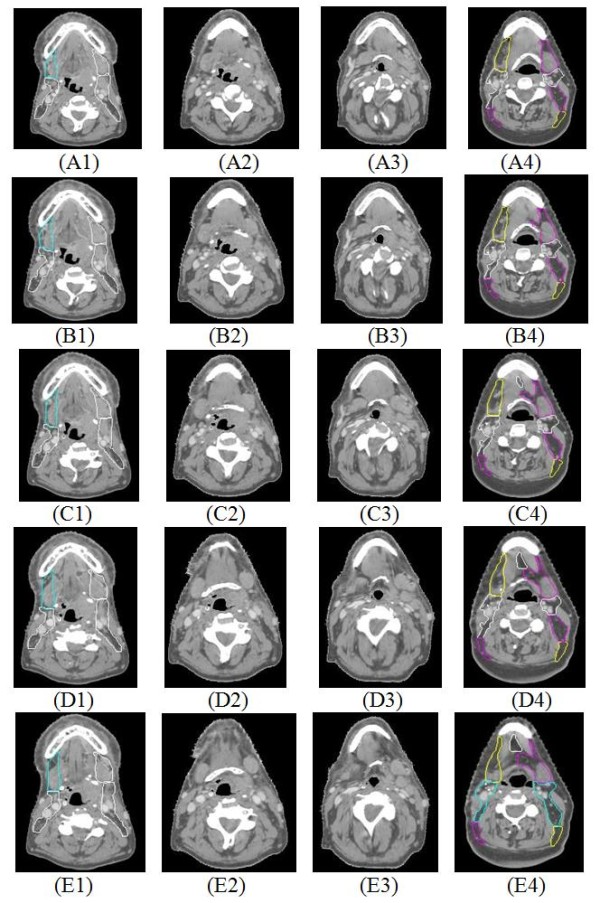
Comparison of results from the image registration methods with and without using landmark correspondence. Rows A-E show selected axial CT slices in the neighborhood of the hyoid in various data sets from superior to inferior. Column 1 shows slices from the reference subject, column 4 from the target subject, column 2 is the result of Mattes' image registration method, and column 3 is the result of the new method using landmark correspondence.
